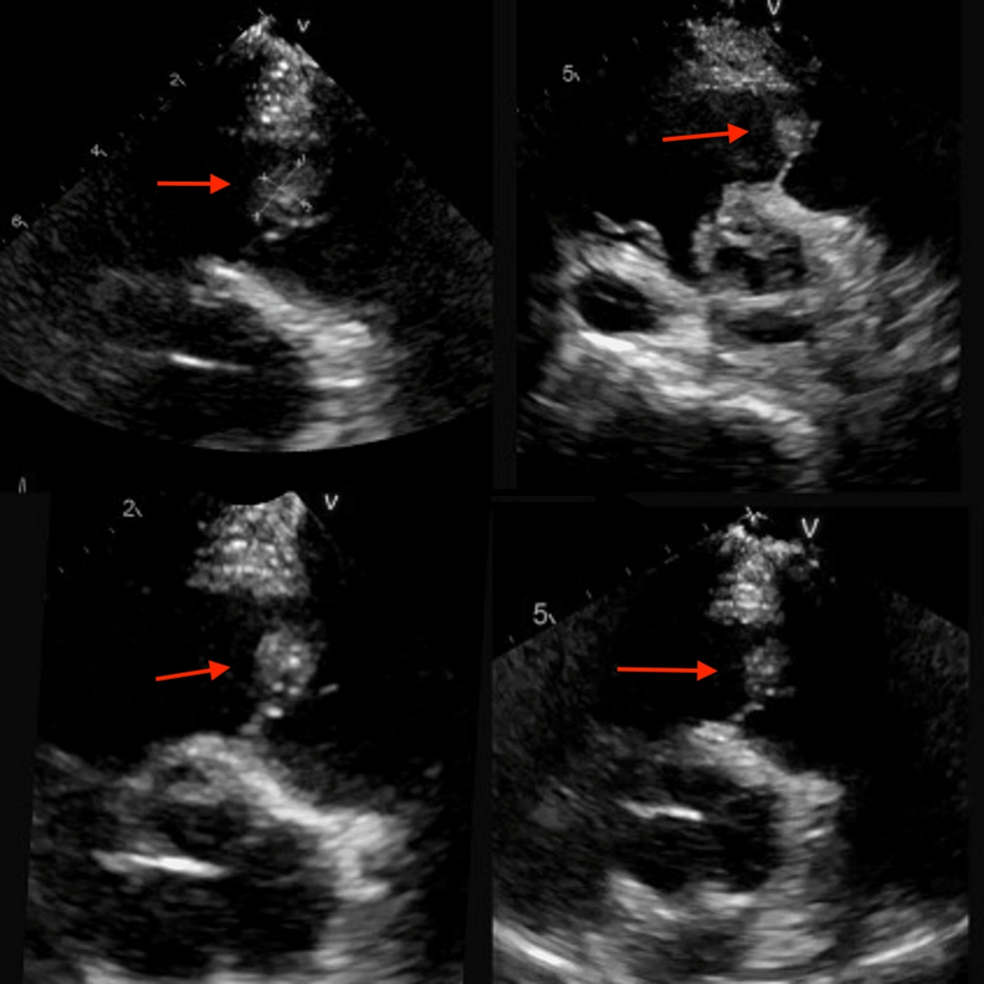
Multiple views from transthoracic echocardiography showing pulmonic valve vegetation measuring 1.29 x 0.89 cm
